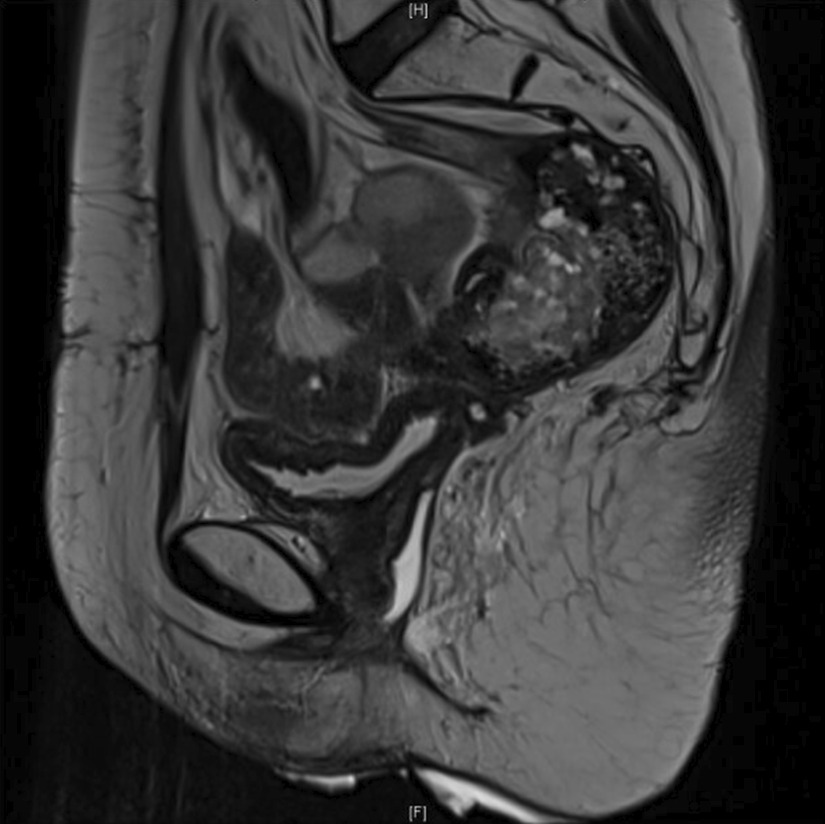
Sagittal pelvic MRI at 6 months follow-up
